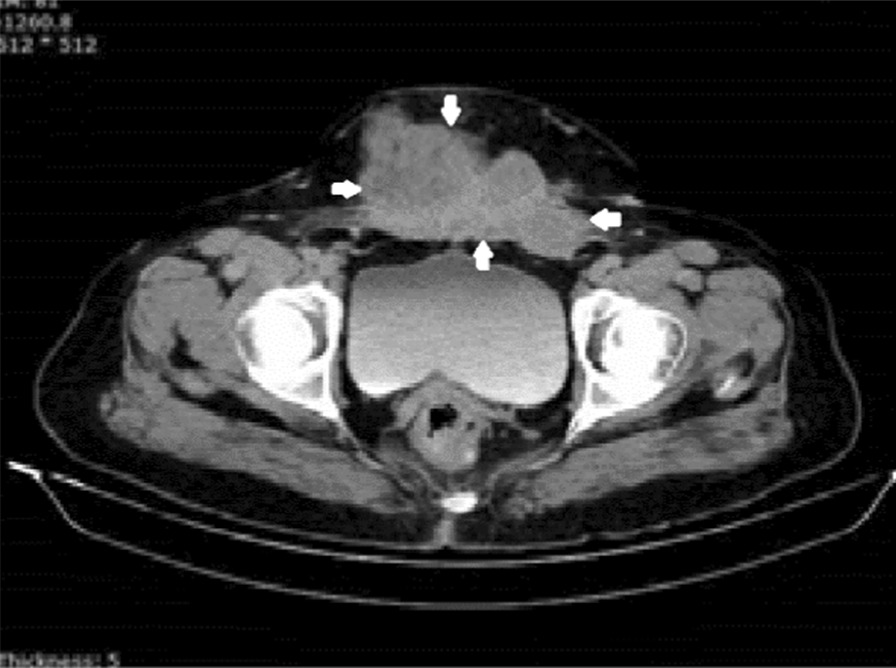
Preoperative pelvic computed tomography scan of patient 1. Borders of the abdominal wall mass are marked by arrows
